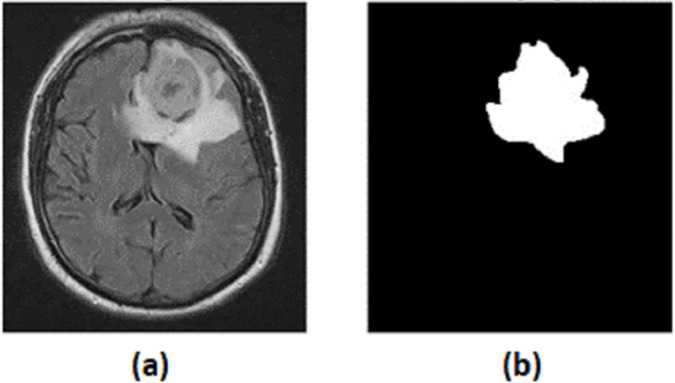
(a) Magnetic resonance image and (b) corresponding binary mask pair.
